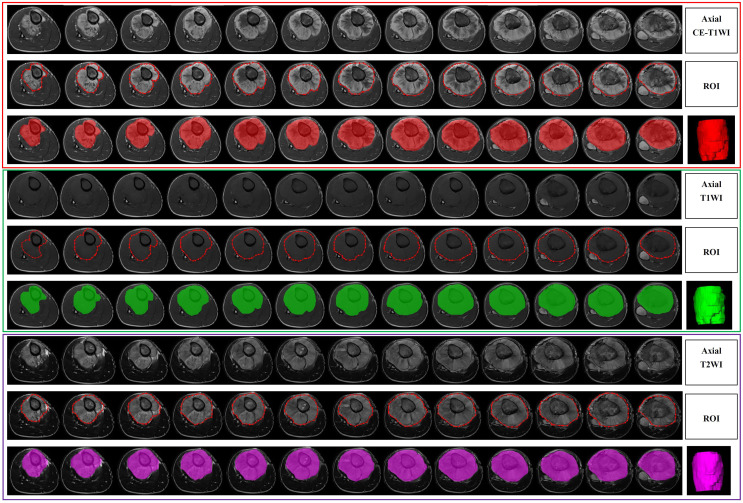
An example of a segmented MRI image.
